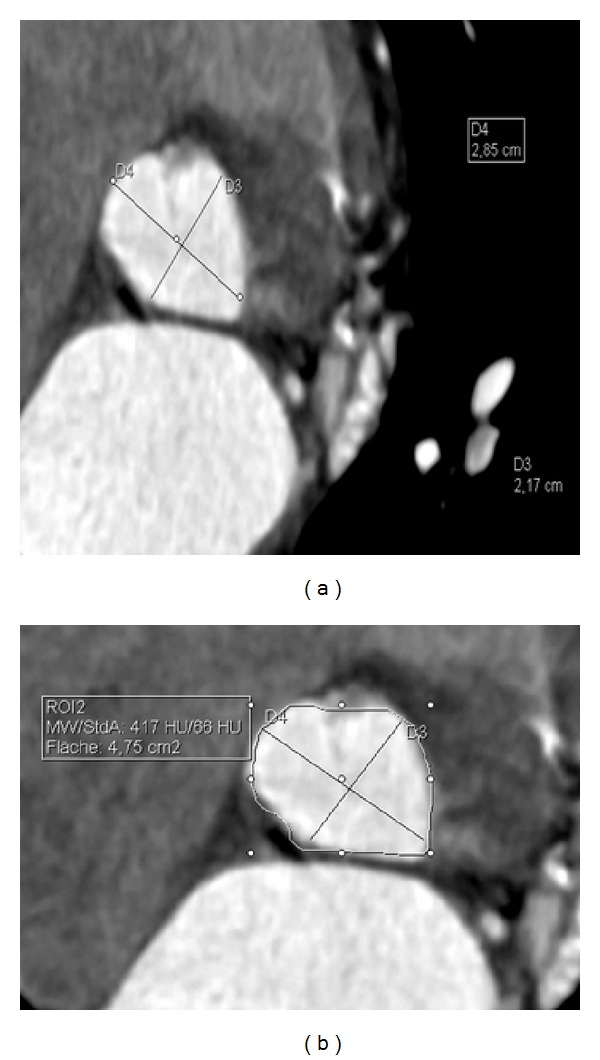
Aortic annulus sizing by CT.* (a): The anterior-posterior (AP) (D3) and mediolateral diameter (ML) (D4) are measured on cross-sectional images. The mean of both diameters is commonly calculated for selecting the appropriate size of the transcatheter aortic valve prosthesis device finally used for TAVI (currently, 23 mm, 26 mm, or 29 mm devices). Exact fit is crucial to avoid paravalvular leakage and to ensure seamless adaption of the prosthesis with the aortic annulus. (b): Quantification of the annulus area is another valuable parameter for selection of the final valve size. Aortic annulus area is traced with a digital caliper (Area, 475 mm^2^).
